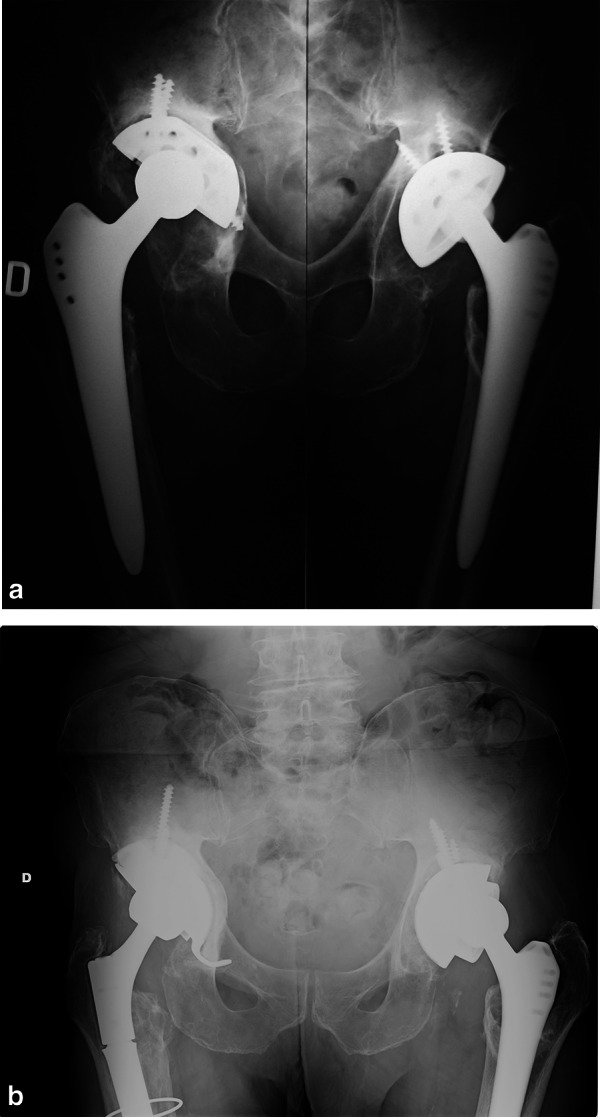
a Preoperative x-rays of bilateral cup aseptic loosening; b 9 years follow-up on right side with Delta TT Revision cup + augment and 8 years follow-up on left side using Delta One TT + face changer
